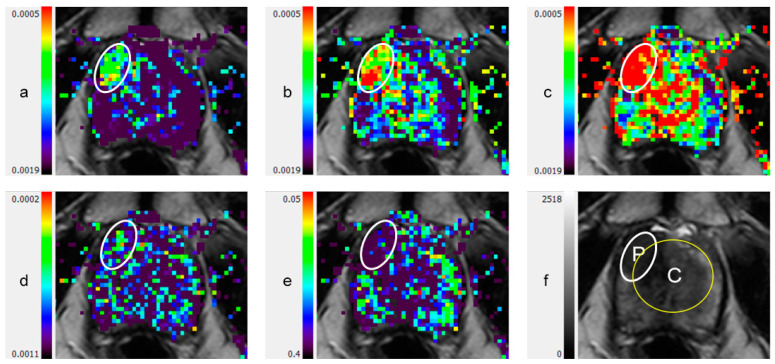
Anterior lesion involving peripheral zone and central gland, on color coded DTI maps and T2-weighted image: (a) λ_1_; (b): λ_2_; (c) λ_3_; (d) maximal anisotropy (MA); (e) fractional anisotropy (FA); (f) T2-weighted image. C: central gland (transitional zone and central zone); DTI: diffusion tensor imaging; P: peripheral zone; White ellipsoid in (a) to (e): anterior lesion.
